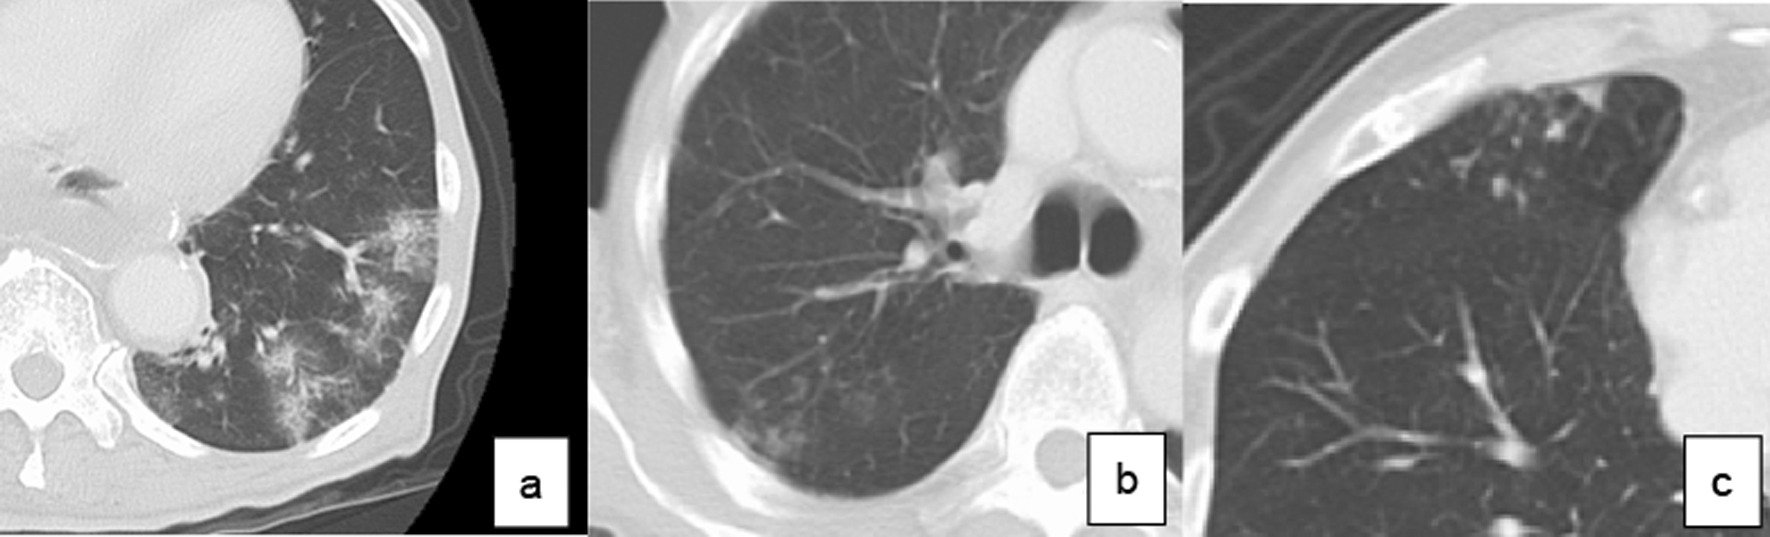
Definition of the diagnosis of pneumonia based on CT a consolidation type, b reticular type, c nodular type
